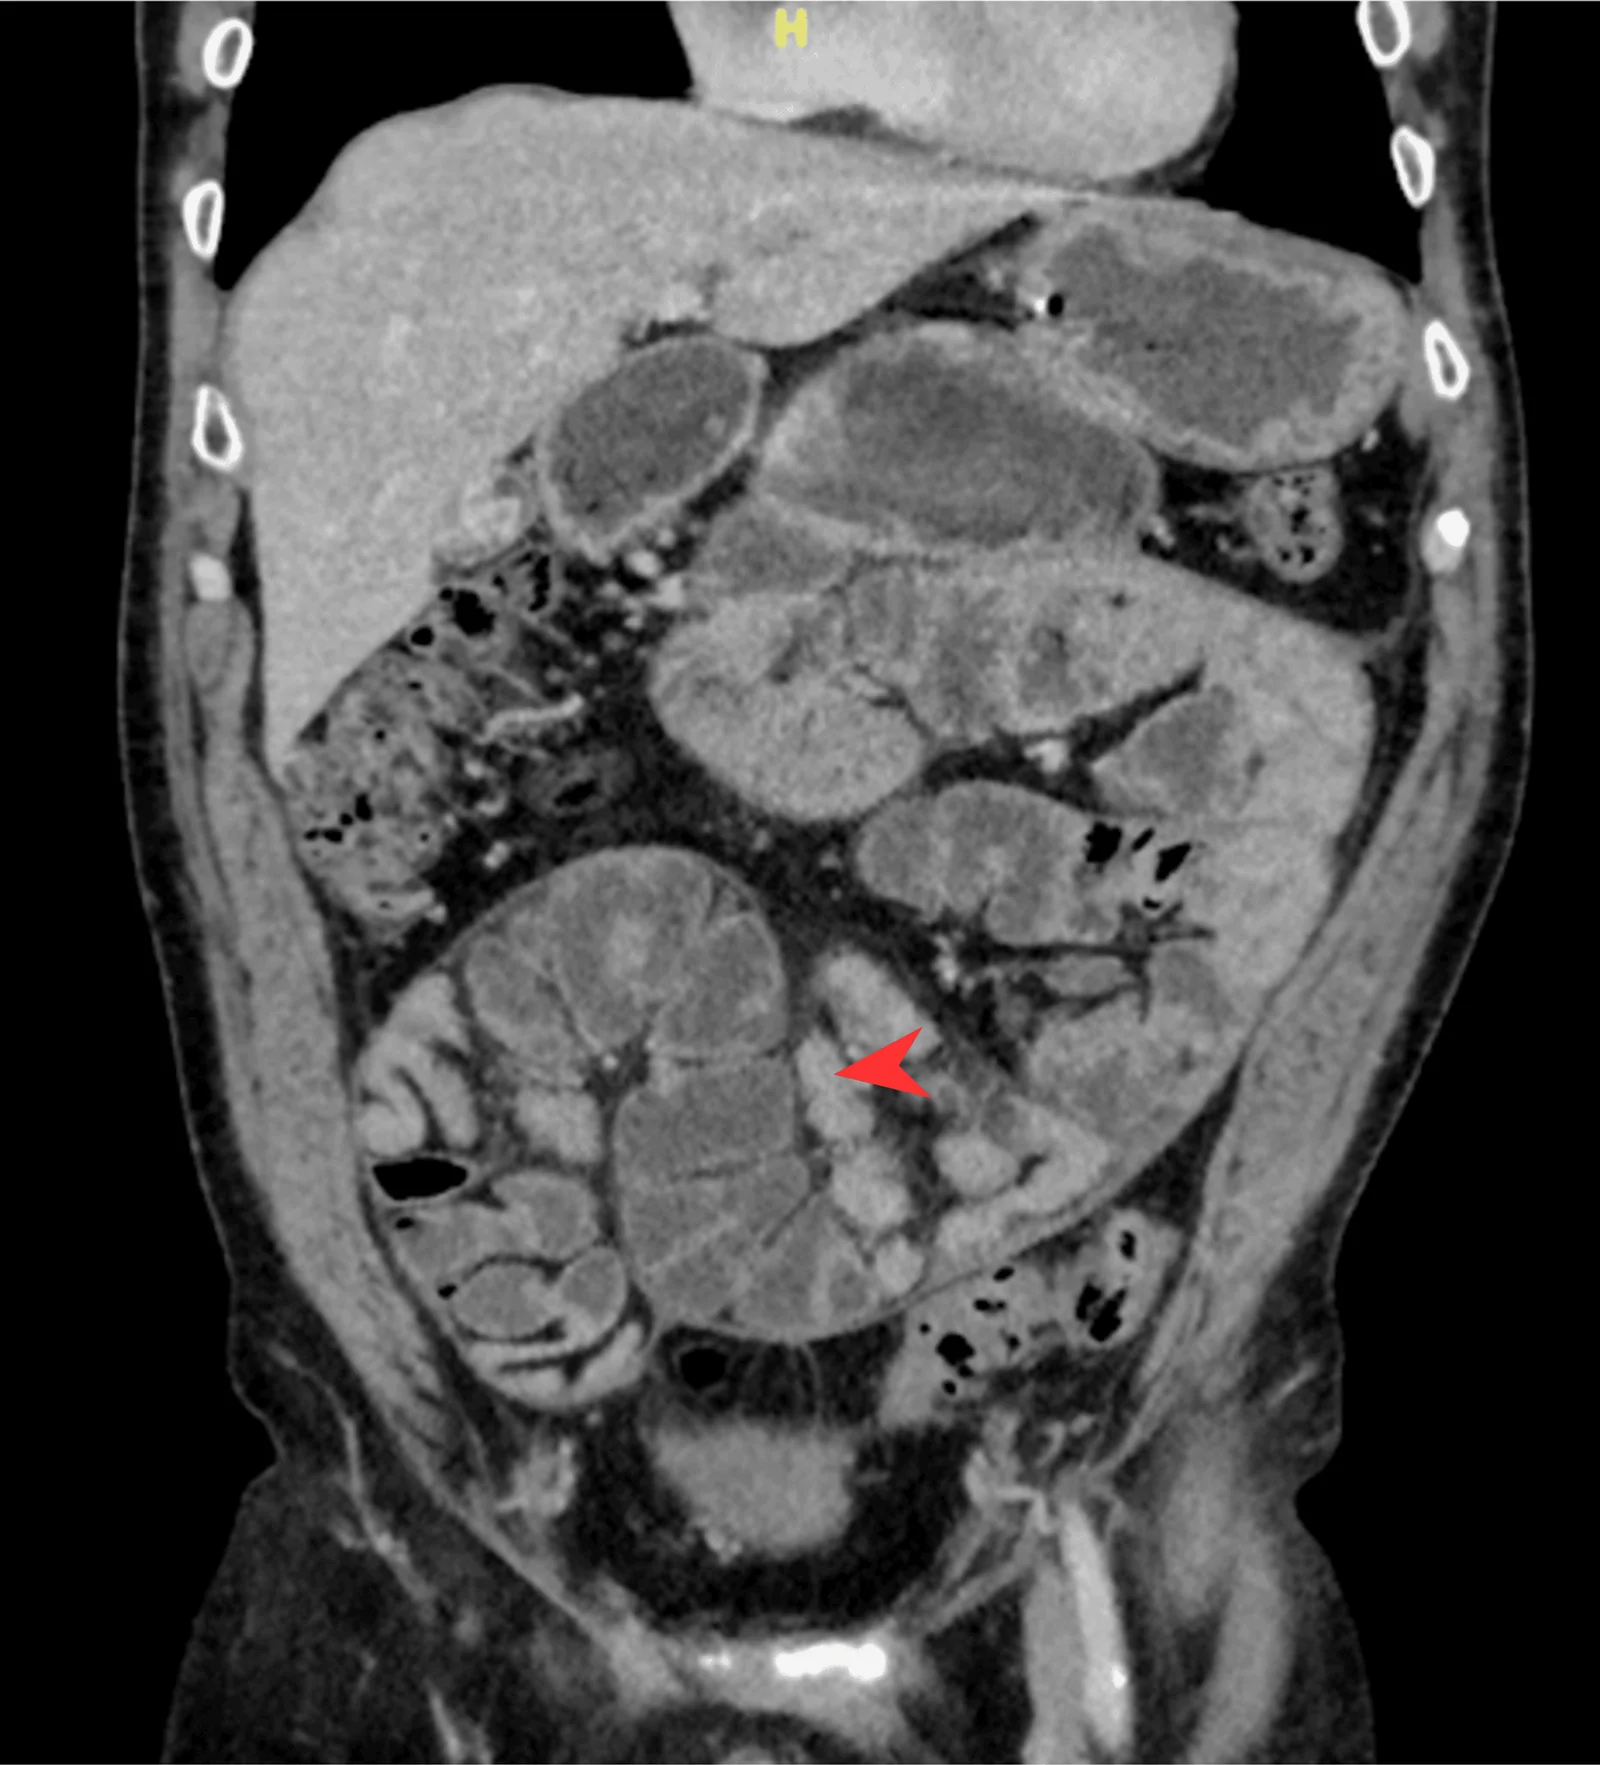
Contrast-enhanced CT of the abdomen and pelvis, coronal view. Significant dilation of multiple small bowel loops is observed, indicative of high-grade intestinal sub-occlusion primarily localized in the right upper quadrant. Additionally, a notable clustered distribution of bowel loops is evident within the central abdomen and the right iliac fossa, indicated by a red arrow.
